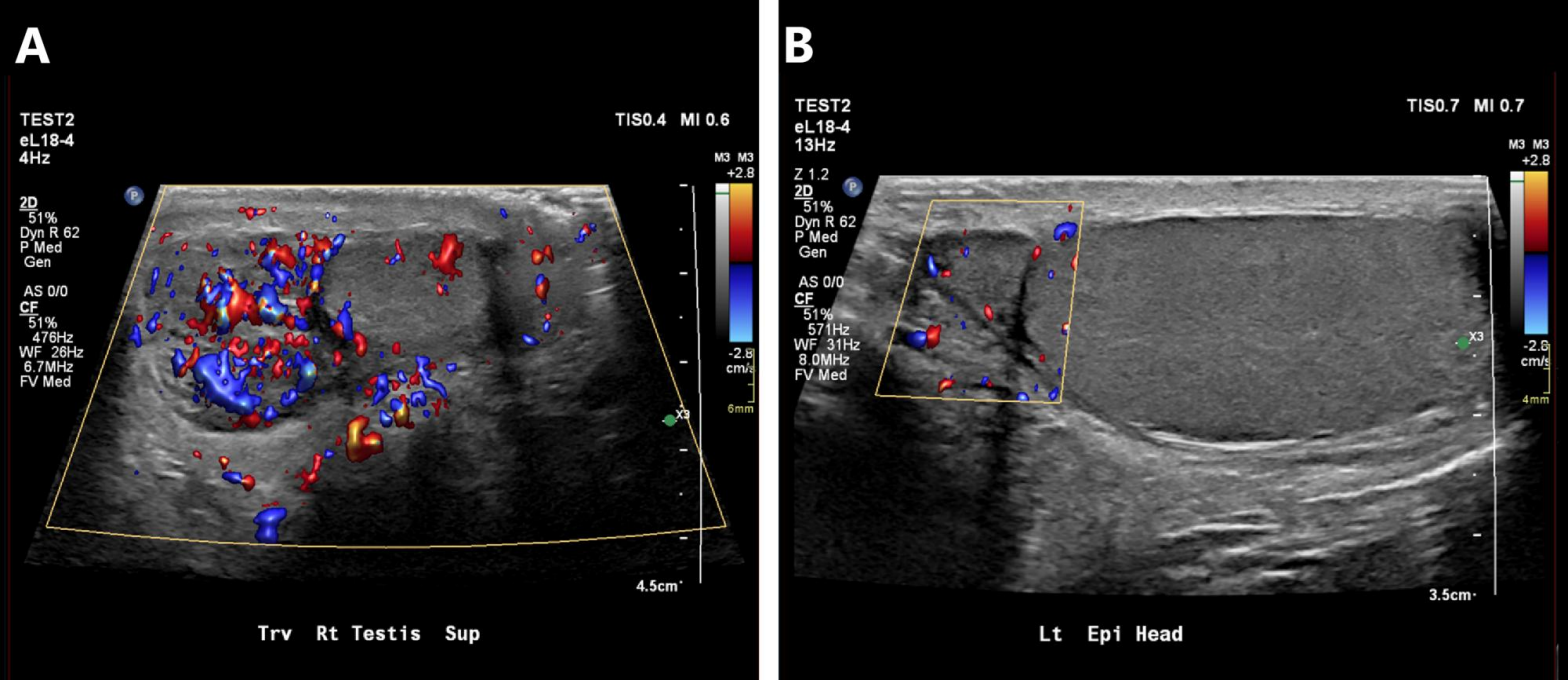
Right-sided epididymitis. (A) The right epididymis is enlarged and heterogeneous testis with marked hyperemia on Doppler interrogation. (B) For comparison, the contralateral epididymis is more normal in size and blood flow.
